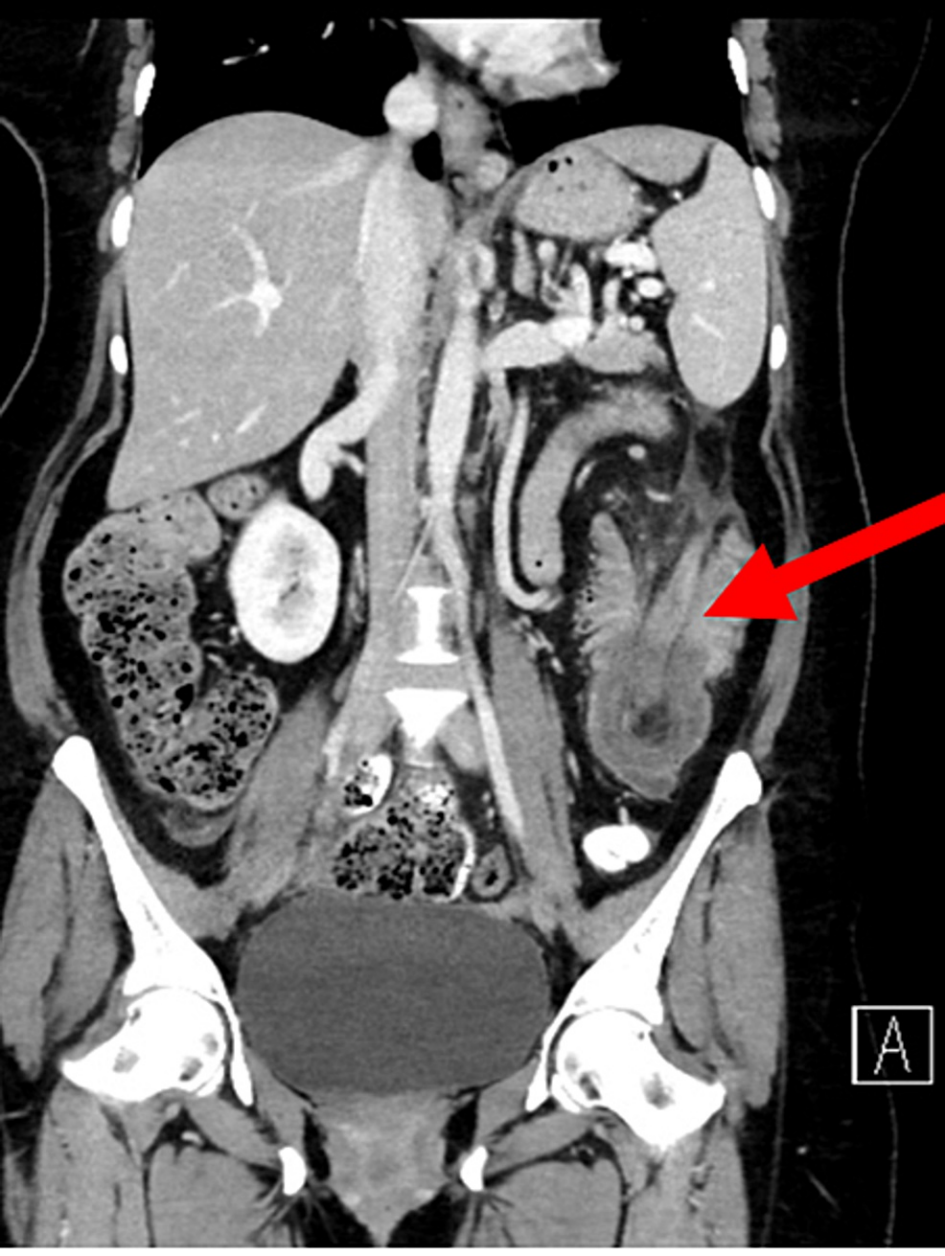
Coronal CT scan showing intussusception
